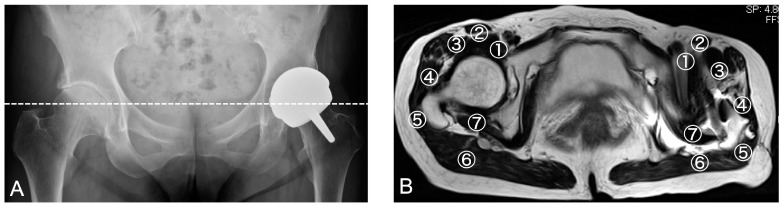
Representative hip resurfacing case assessed using X-ray and MRI. Apparent complications in this representative patient were not found by hip X-ray (A). The periprosthetic muscles consisted of 1. iliopsoas, 2. sartorius, 3. rectus femoris, 4. gluteus minimus, 5. gluteus midius, 6. gluteus maximus, and 7. short rotators which were observed by axial MRI (B) from the ASIS to lessor trochanteric level on T1 and T2 STIR images. If the contours of the targeting muscles cannot be observed, the muscle is regarded as unassessable.
